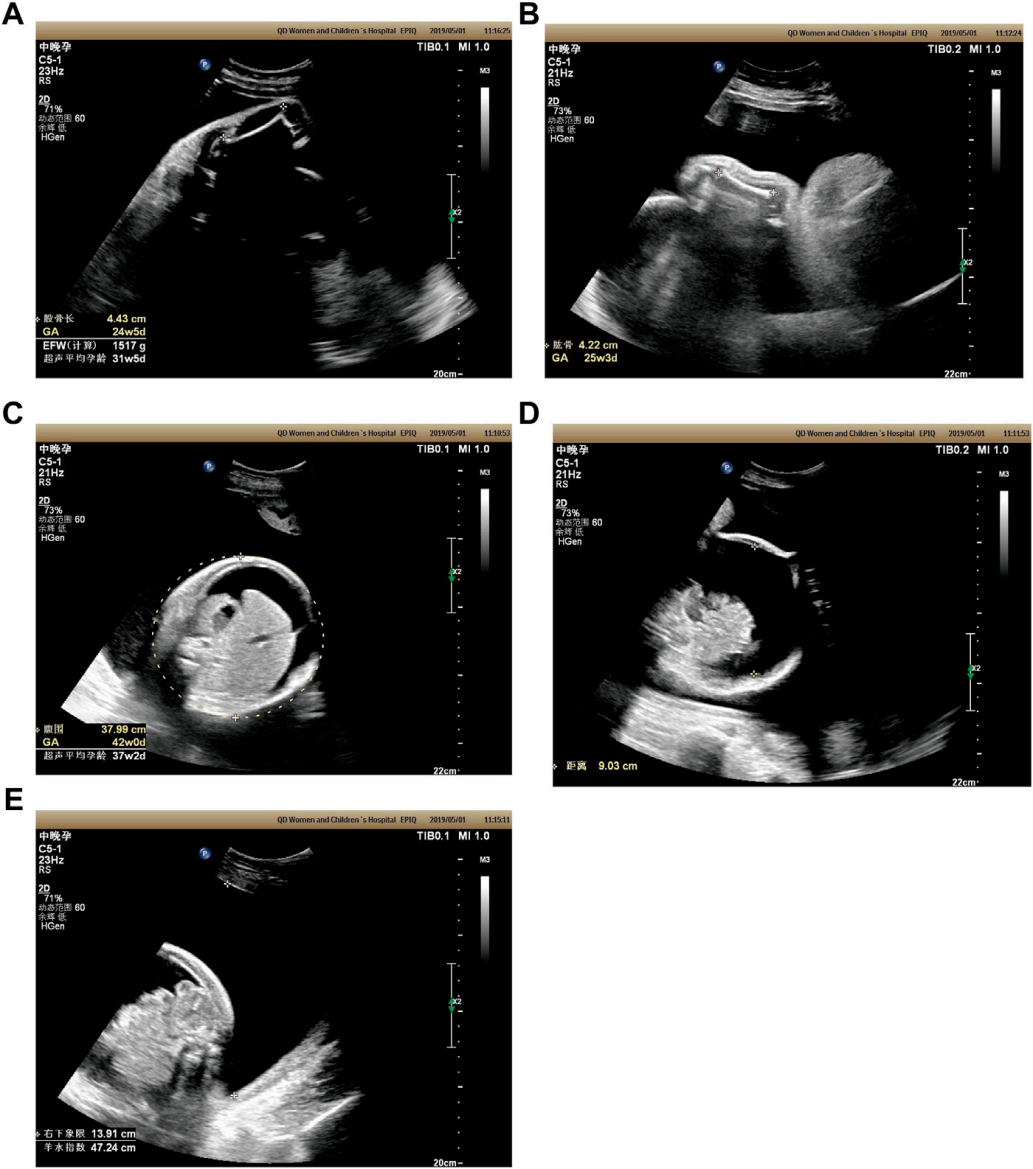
Antenatal ultrasound of foetus 2 (A) The femur length was 4.4 cm. (B) The humerus length was 4.2 cm. (C)The abdominal circumference was 38.0 cm. (D) The seroperitoneum was 9.0 cm. (E)The amniotic fluid index was 47.0 cm.
